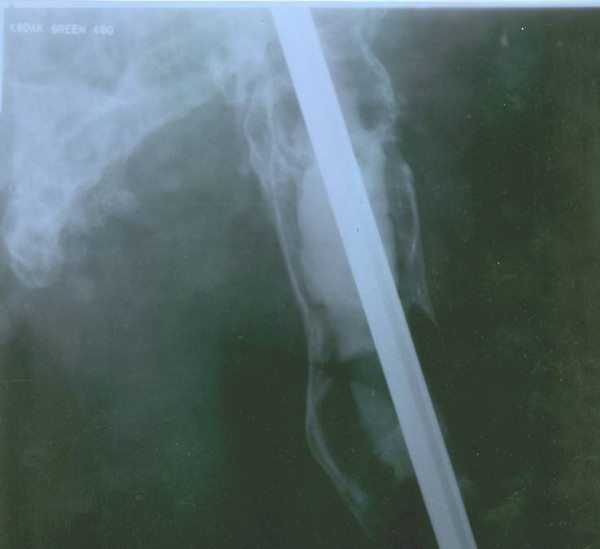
Plain x-ray of right femur showed advanced lytic destruction of bone, centered at the proximal two thirds of the right femur. There is also a nailing due to previous surgery.
